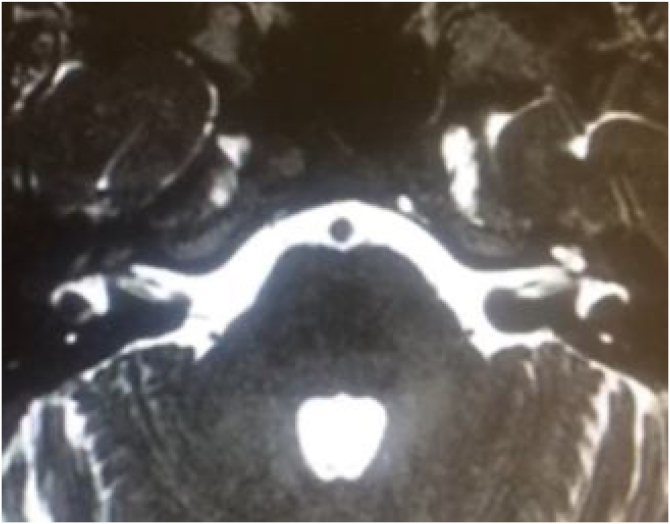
Magnetic resonance imaging (T2-weighted image). Axial plane. Patient with meningitis. Ossification of the cochlea on the right side.
